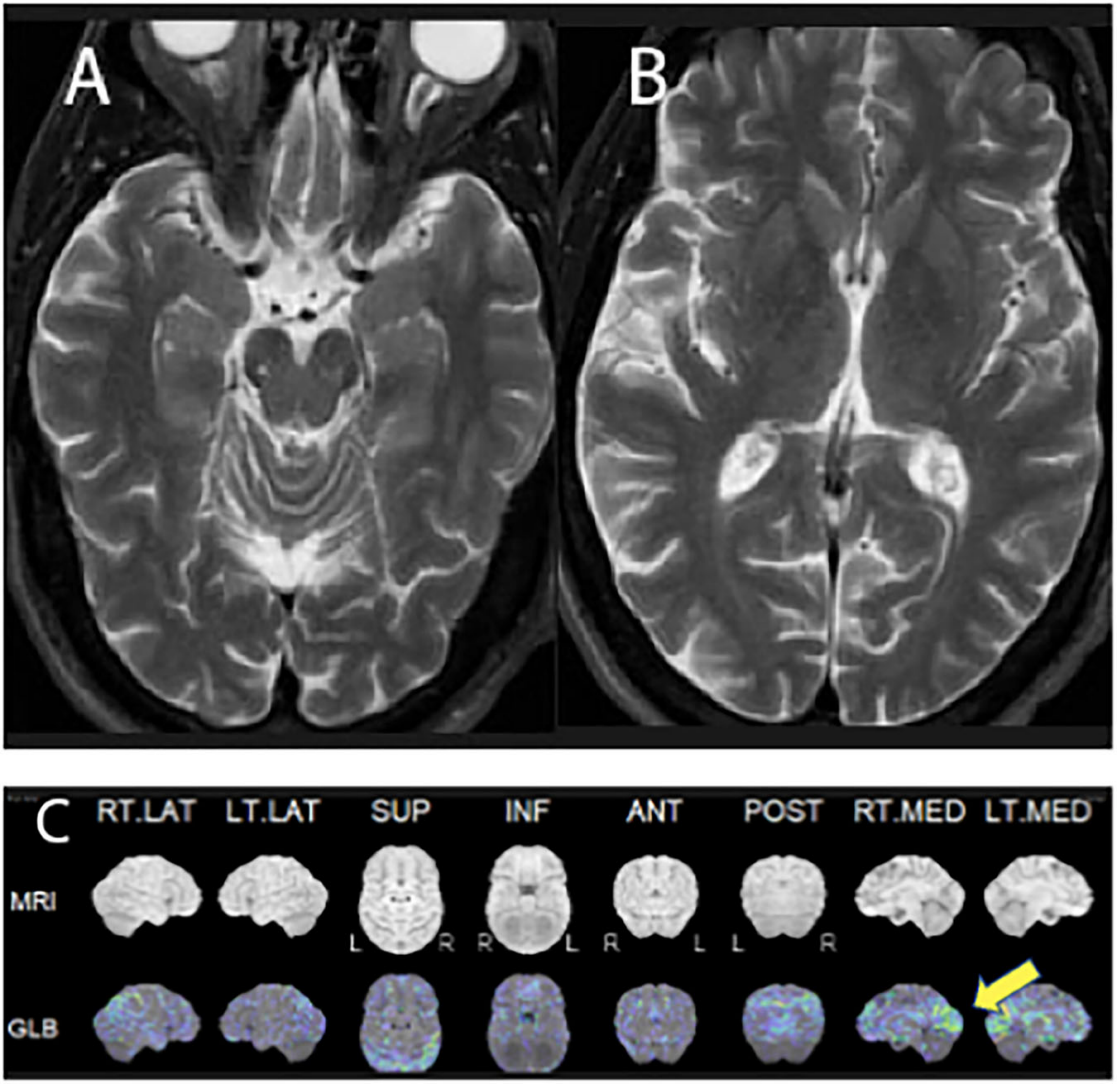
70-year-old male presents with a 1-year history of cognitive dysfunction, loss of executive function, diplopia, brain fog, short-term memory loss, disorientation, and REM sleep behavioral disturbance. T2-weighted axial image of the hippocampus (A) and the basal ganglia (B) demonstrates normal hippocampal volume; temporal and frontal lobes are unremarkable. FDG-PET with 3D-SSP reconstruction (C) shows profound occipital hypometabolism, right greater than left, but the posterior cingulate is preserved. The findings are consistent with dementia with Lewy body (DLB)
